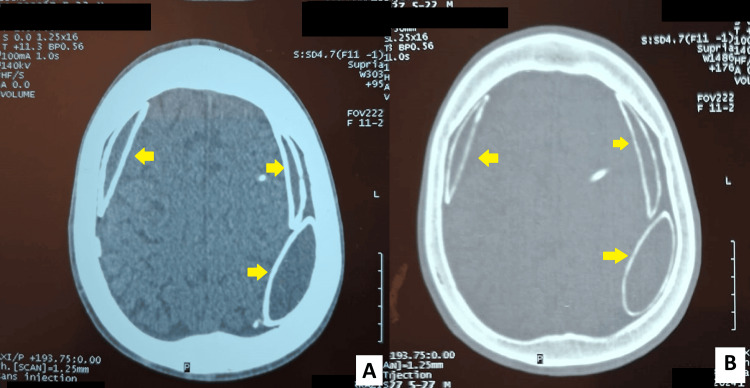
Control CT scan at two years follow-up showing a stable aspect of the collections. (A) Parenchymal window showing persistent hypodense organized calcified subdural collections (yellow arrows). (B) Bone window emphasizing dense laminar calcification along the convexities (yellow arrows).
